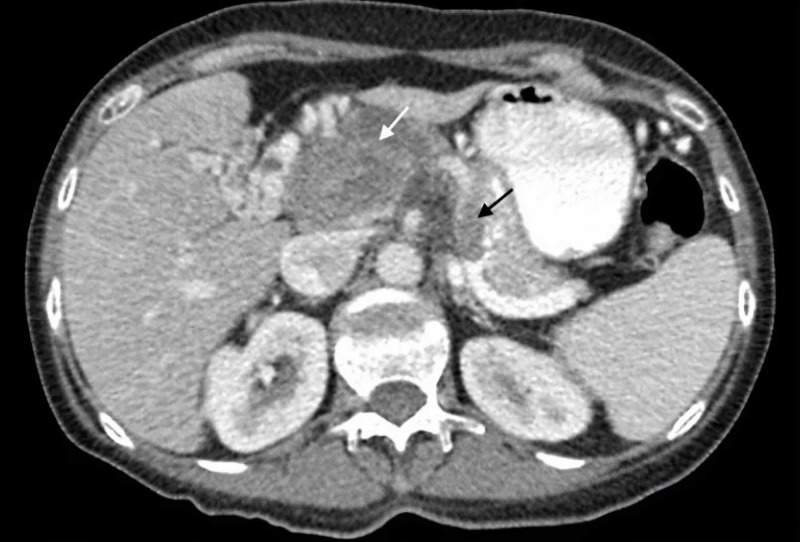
Axial plane abdominal contrast-enhanced CT showing a mass lesion (white arrow) adjacent to liver and aorta, and a low attenuation filling defect in the lumen of splenic vein (black arrow).
